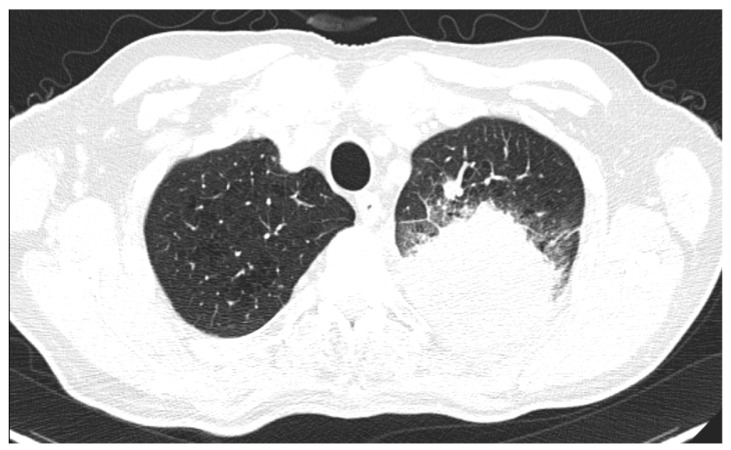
Axial CT lung window view of a spiculated ADC tumour localised in the left pulmonary apex.
